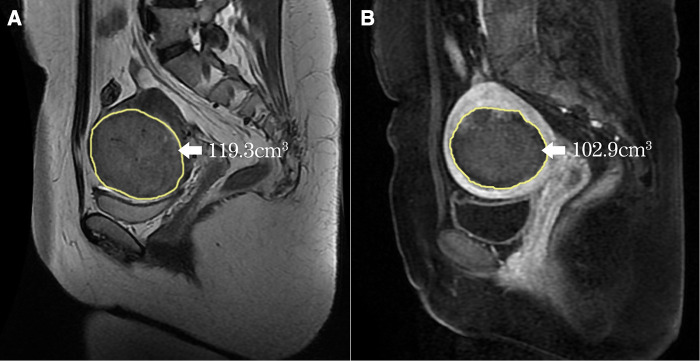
Measurement of the volume of the fibroid and the NPV (yellow line). (A) The fibroid was outlined on every slice of MRI, the automatically generated volume was 119.3 cm^3^; (B) The NPV was also outlined on every slice of CE-T1WI, the automatically generated NPV was 102.9 cm^3^.
